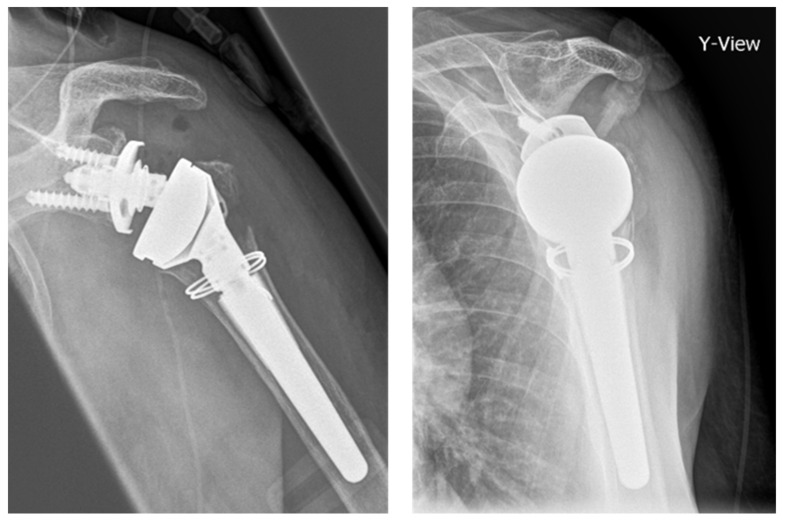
X-rays of the left shoulder of an 82 year-old patient after implantation of a modular, non-cemented proximal humeral fracture prosthesis (RSA) with protective cerclage (1.5 mm steel wire) at the metaphyseal shaft.
